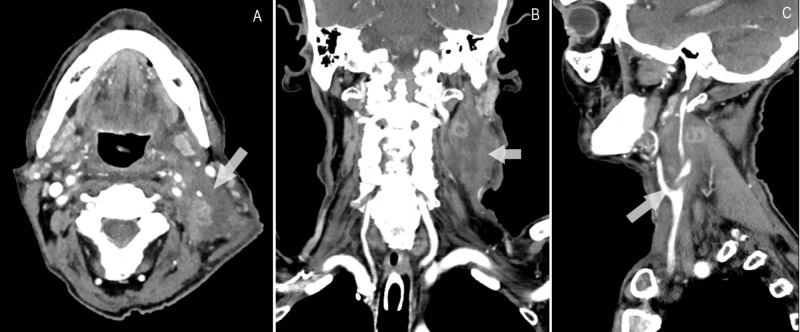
Cervical CT-scan after radiotherapy, showing significant decrease in mass size (A) Axial view; (B) coronal view; (C) sagittal view.
